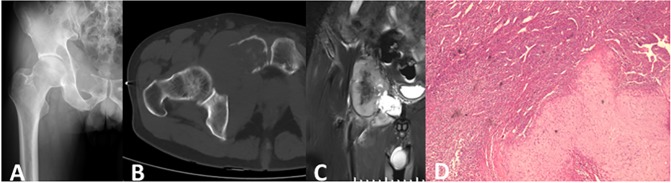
Dedifferentiated chondrosarcoma of the right pelvis in a 58-year-old man (Case 15). (A). Anteroposterior radiograph demonstrated osteolytic bone destruction and a soft tissue mass without chondral or osteoid matrix mineralization. (B). CT showed punctate chondral matrix mineralization in the intraosseous medullary cavity, which might be high-grade chondrosarcoma. (C). Fat-suppressed coronal T2-weighted image showed a clear demarcation between predominant reduced signal intensity (dedifferentiated component) and chondral signal intensity. (D). Pathological section (HE X40) showed feature of the low grade chondrosarcoma and high grade malignant fibrous histiocytoma.
